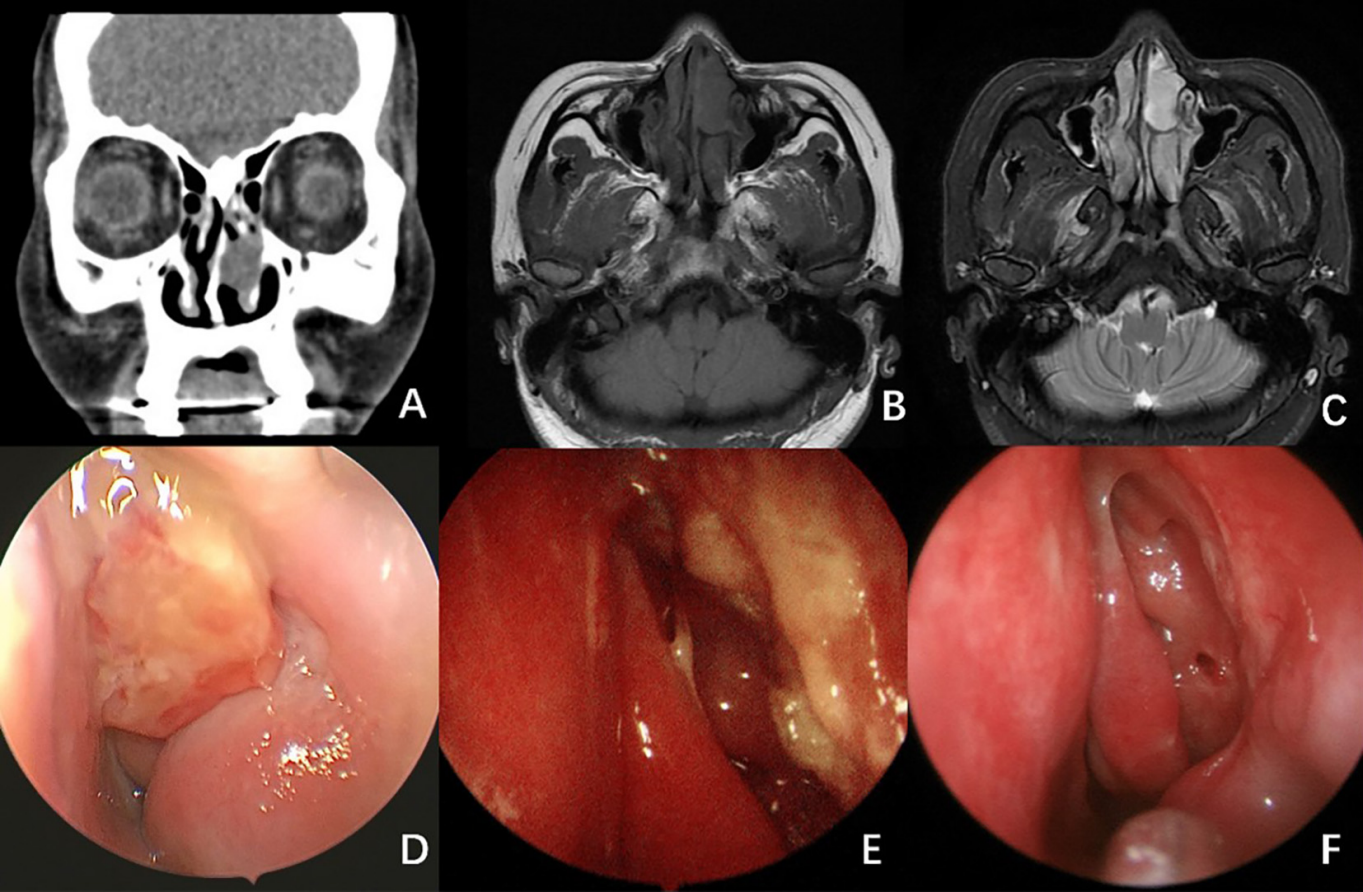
Radiological And Endoscopy Images of Case 2. (A) CT scan of the paranasal sinuses showed a soft tissue density mass in the left nasal cavity. (B, C) MRI scan showed an isointense T1 (B) and hyperintense T2 (C) nodular mass in the left nasal cavity, with relatively clear boundaries and measuring approximately 14 × 23 × 20 mm, exhibiting mostly uniform signals. (D) Endoscopy image revealed a neoplasm in the left nasal passage, characterized by surface vascular dilation and purulent secretions. (E) Endoscopic image 2 months after surgery. (F) Endoscopic image 5 months after surgery. No signs of recurrence were observed.
